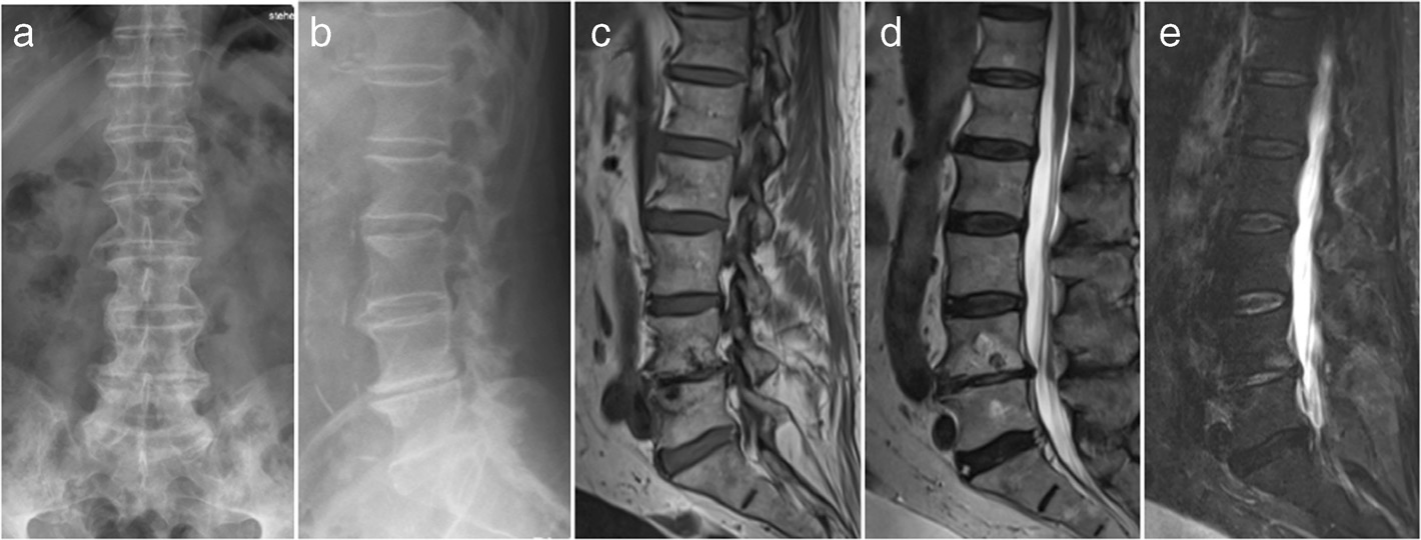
Examplary case of an 81 year old male patient presenting with immobilizing low back pain (VAS 8/10), fever and an elevated CRP serum level of 52 mg/l after 6 weeks of appropriate conservative therapy. Conventional X-ray images upon admission in an upright standing position a.p. (a) and lateral (b) Pre-operative sagittal MR T1-weighted (c) T2-weighted (d) and STIR (e) images demonstrating the bony endplate lesions at the L4/5 level
